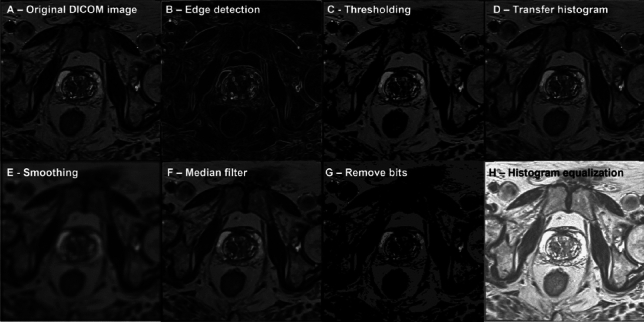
Pre-processing of one MR image. The original images undergo several pre-processing procedures i.e. B-Edge detection, C-Thresholding, D-Transfer histogram, E-Smoothing, F Median filter, G Remove bits and H-Histogram equalisation
